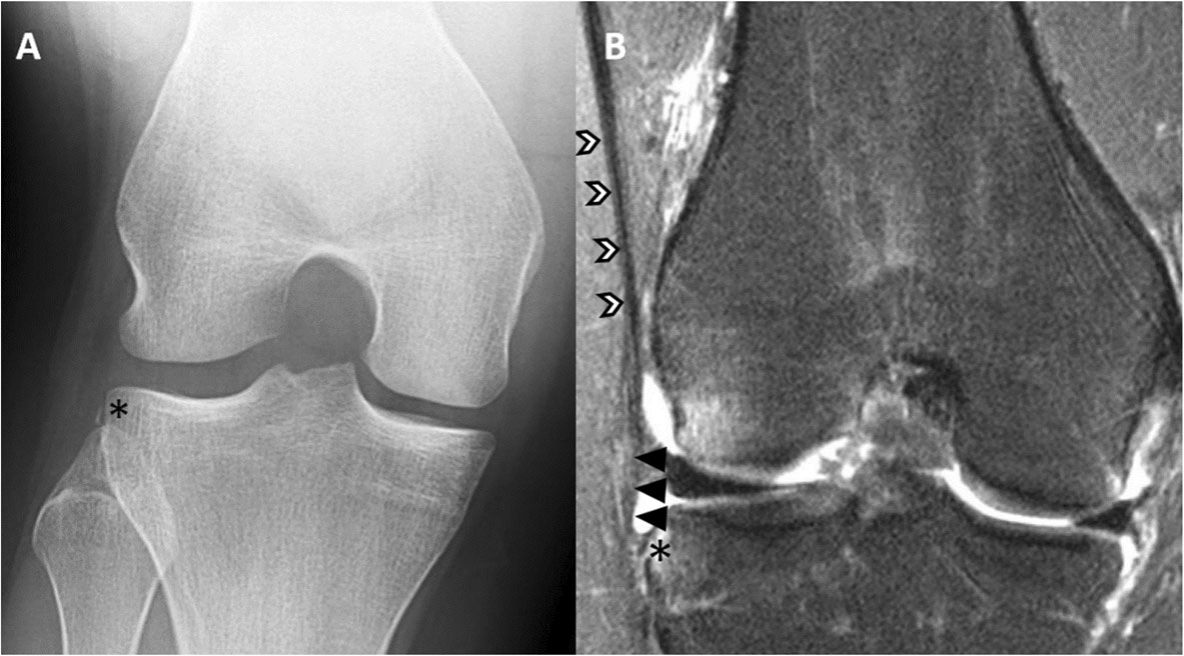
Radiograph (a) and magnetic resonance imaging exam (b) of a Segond fracture with injury to the anterolateral capsule. The black star denotes the Segond fracture, or an avulsion fracture off the lateral tibial plateau. The black and white errors denote the iliotibial band, while the black arrows demonstrate the anterolateral capsule
